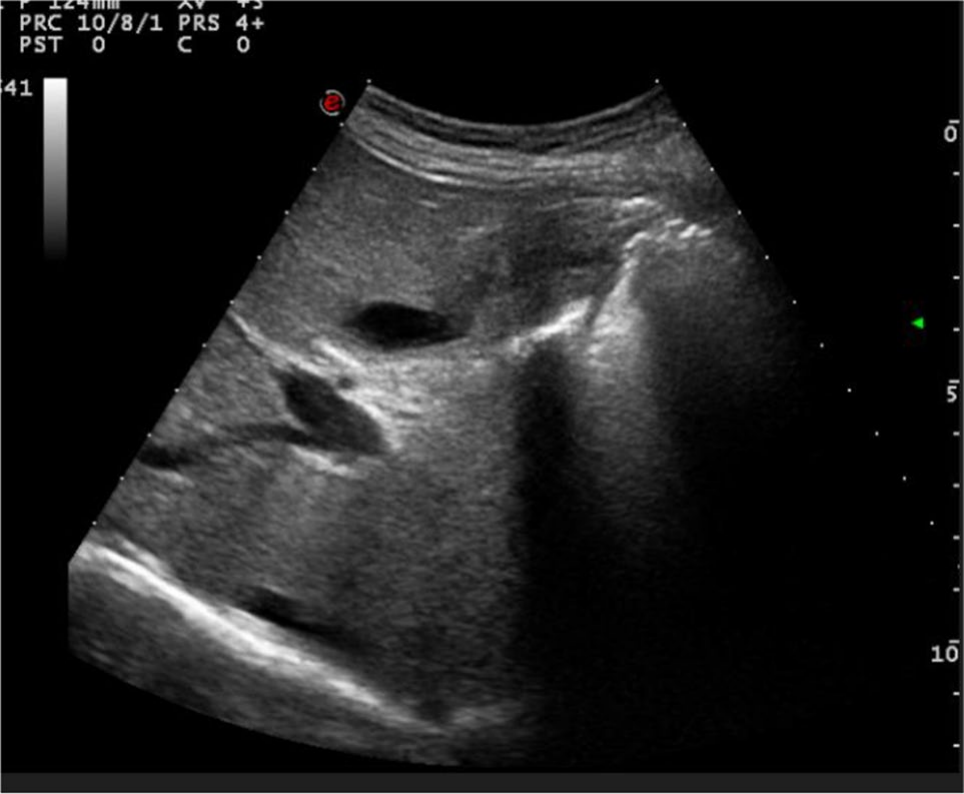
B-mode ultrasound of the gallbladder showing pathologic wall thickening (14 mm) localized in the body and fundus of the gallbladder, with no evidence of gallstones
